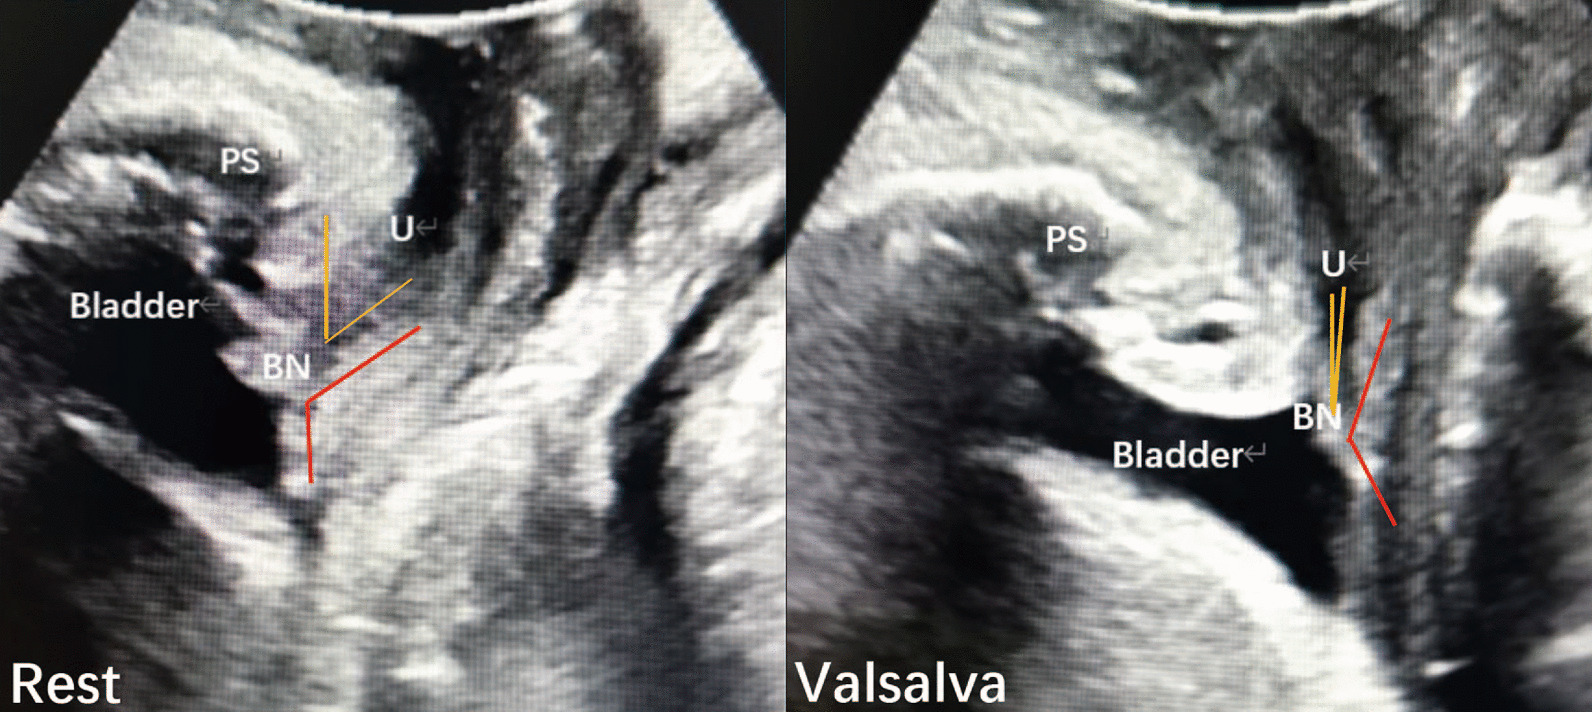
Perineal ultrasoubd image of the pelvic organs. Anatomical landmarks are the pubic symphysis (PS) and bladder with bladder neck (BN). The urethra (U). The posterior urethrovesical angle (the red arrow) and urethral inclination angle (the yellow arrow) was measured both at rest and on maximal Valsalva manoeuver
